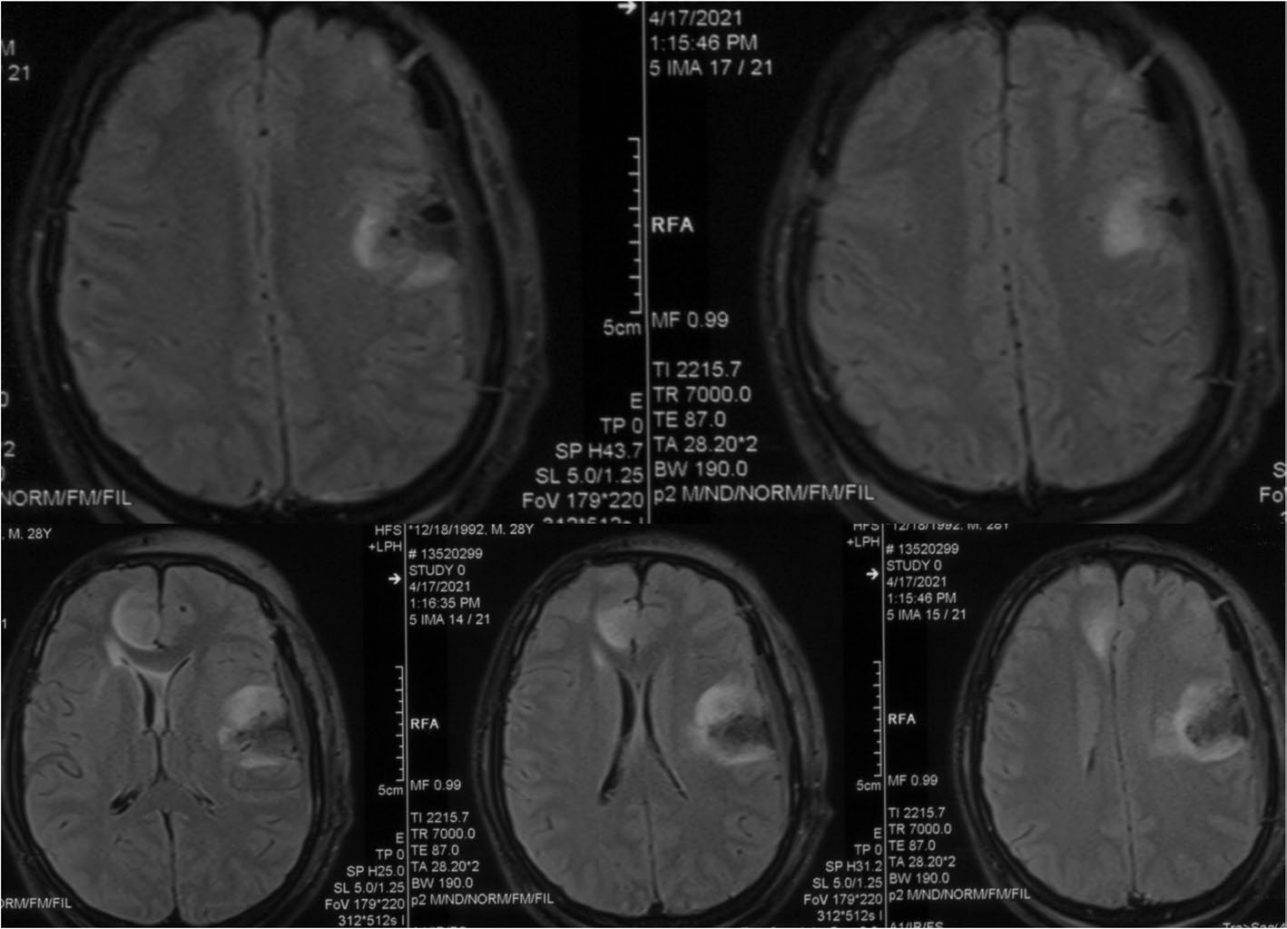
Postoperative magnetic resonance image (axial T2 fluid-attenuated inversion recovery)
